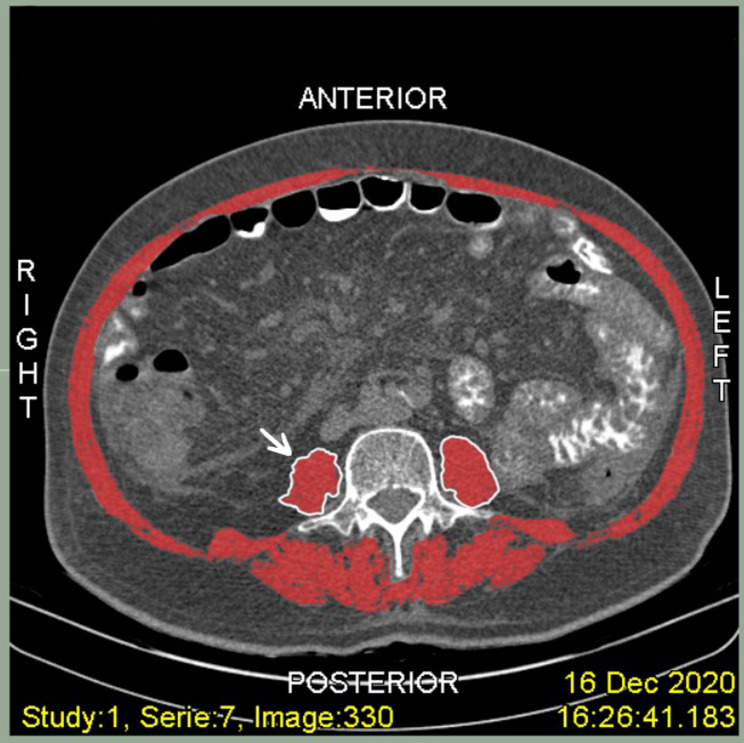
Representative axial CT image at the level of L3 demonstrating skeletal muscle segmentation for calculation of SMI. The highlighted regions (in red) represent the total skeletal muscle area, including the psoas, paraspinal, and abdominal wall muscles, identified using standardized Hounsfield unit (HU) thresholds for muscle tissue (− 29 to + 150 HU). Segmentation was performed using a semi-automated approach and subsequently verified by two independent blinded assessors. The psoas muscles are shown bilaterally adjacent to the vertebral body for anatomical reference
